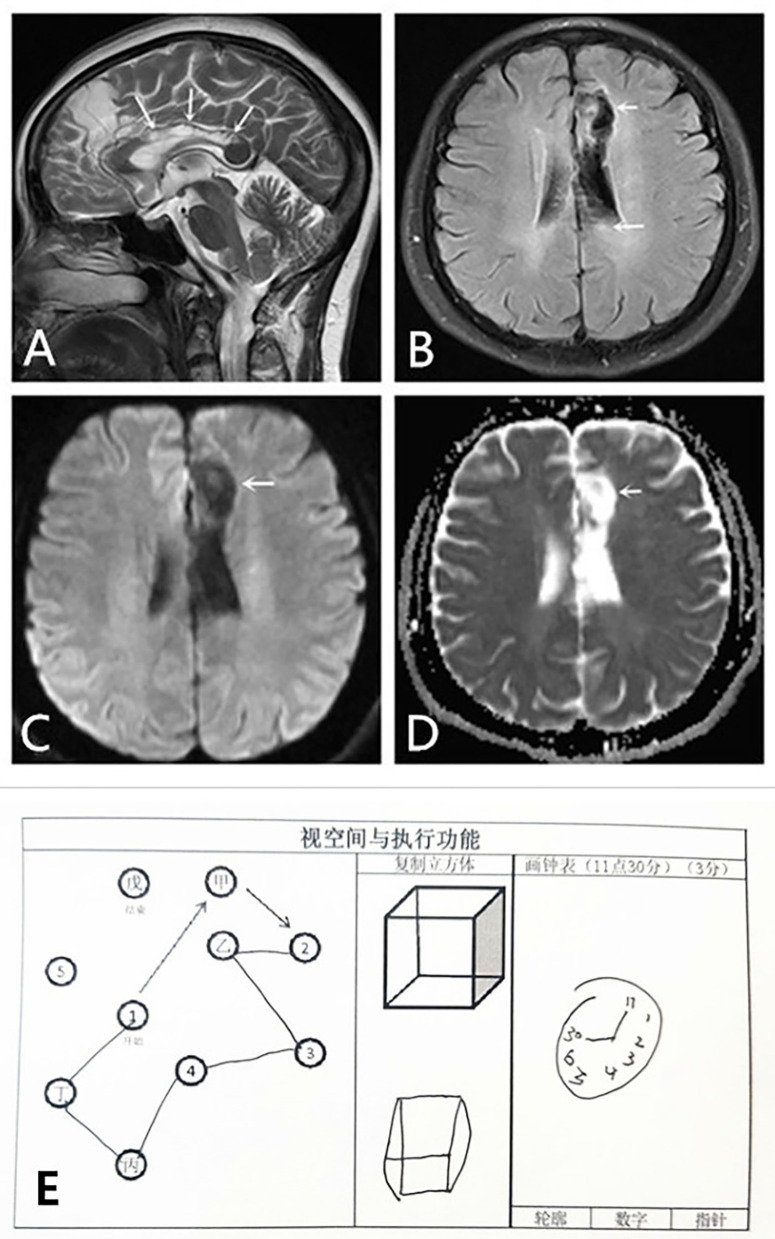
Cytotoxic edema and visuospatial and executing skills of the dominant hand of the patient improved in 3 months. MRI: (A,B) Sagittal T2WI scan and axial T2 FLAIR show obvious improvement of cytotoxic edema in the left frontal lobe and the body of the corpus callosum. Encephalomalacia foci and gliocyte hyperplasia can be observed in these areas. (C,D) DWI and ADC scans show no limitation of diffusion. The patient failed to recognize spatial structure; however, she has made some progress after this period of recovery (E). ADC, apparent diffusion coefficient; DWI, diffusion-weighted imaging; FLAIR, fluid-attenuated inversion recovery; MRI, magnetic resonance imaging; T2WI, T2-weighted imaging.
